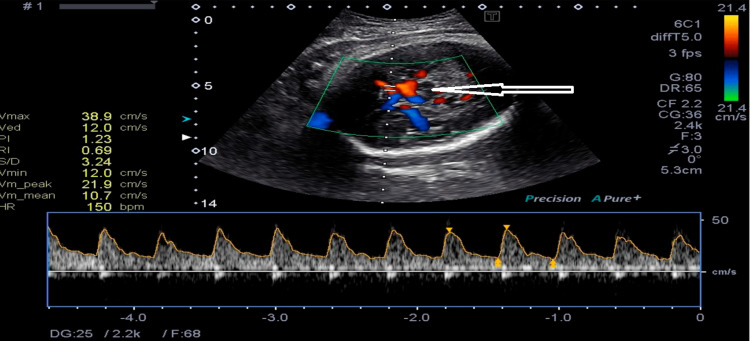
Doppler imaging of cerebral blood flow through MCA (arrow) showing changes at 33 weeks of GA in HIE. MCA: middle cerebral artery; HIE: hypoxic-ischemic encephalopathy; GA: gestational age.
